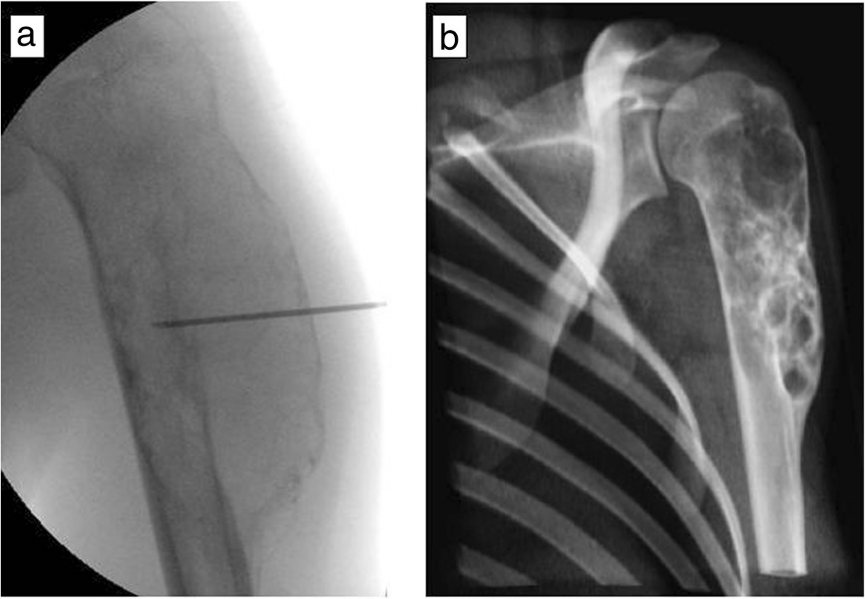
Fluoroscopy picture taken during the 5th injection of polidocanol, showing proper placement of the needle and signs of sclerosis (a), and graphical reconstruction of a computed tomography scan of the proximal humerus, 18 months after the last treatment, showing consolidation of the lesion (b).
